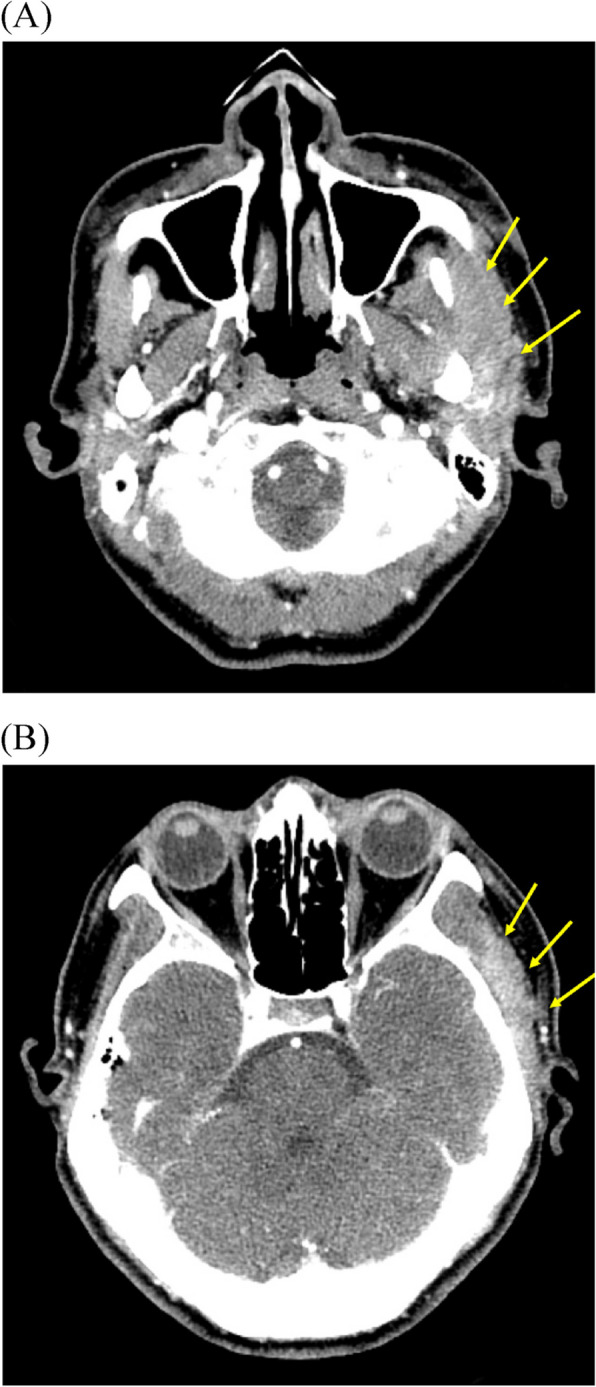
Neck angiography CT images 2 months after the first visit (case 1). Heterogeneous lesions of left masseteric and temporal space (yellow arrows) were decreased but remained. A Masseteric space. B Temporal space
